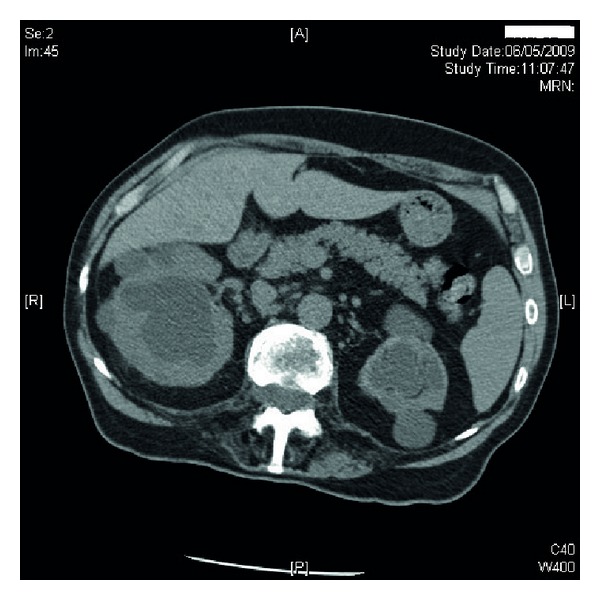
Computed tomography of abdomen revealed marked right hydronephrosis and mild-to-moderate left hydronephrosis. There were several large simple cortical cysts bilaterally, and there was also cortical thinning bilaterally.
